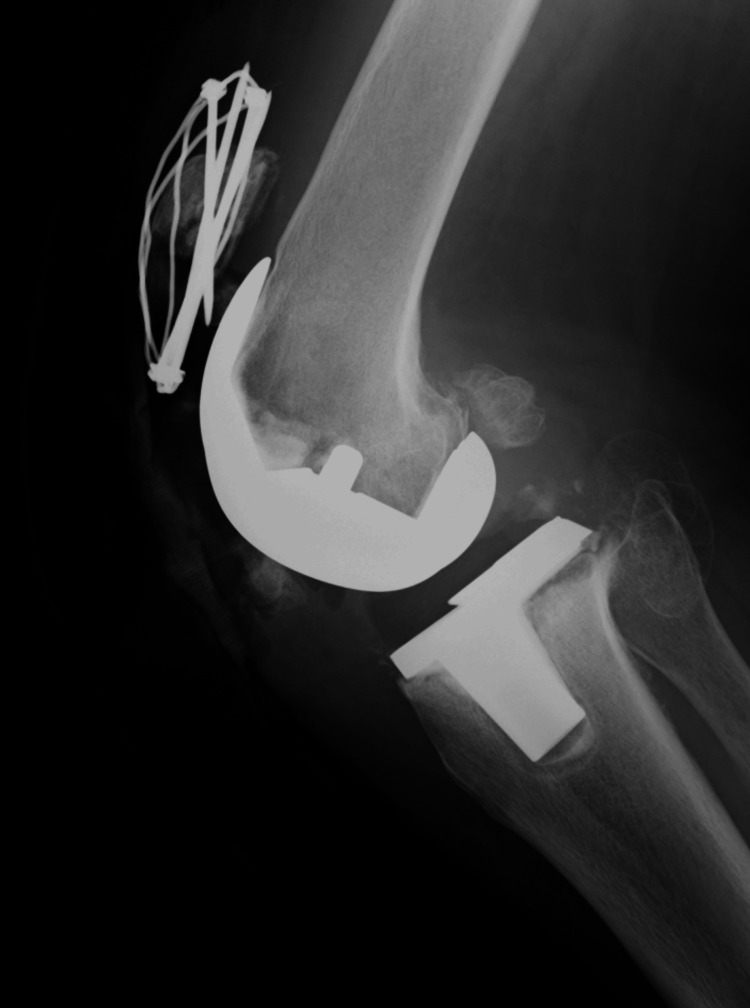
X-ray before implant removal Due to the inability to control the infection with debridement, bone union was not achieved, resulting in implant loosening.
